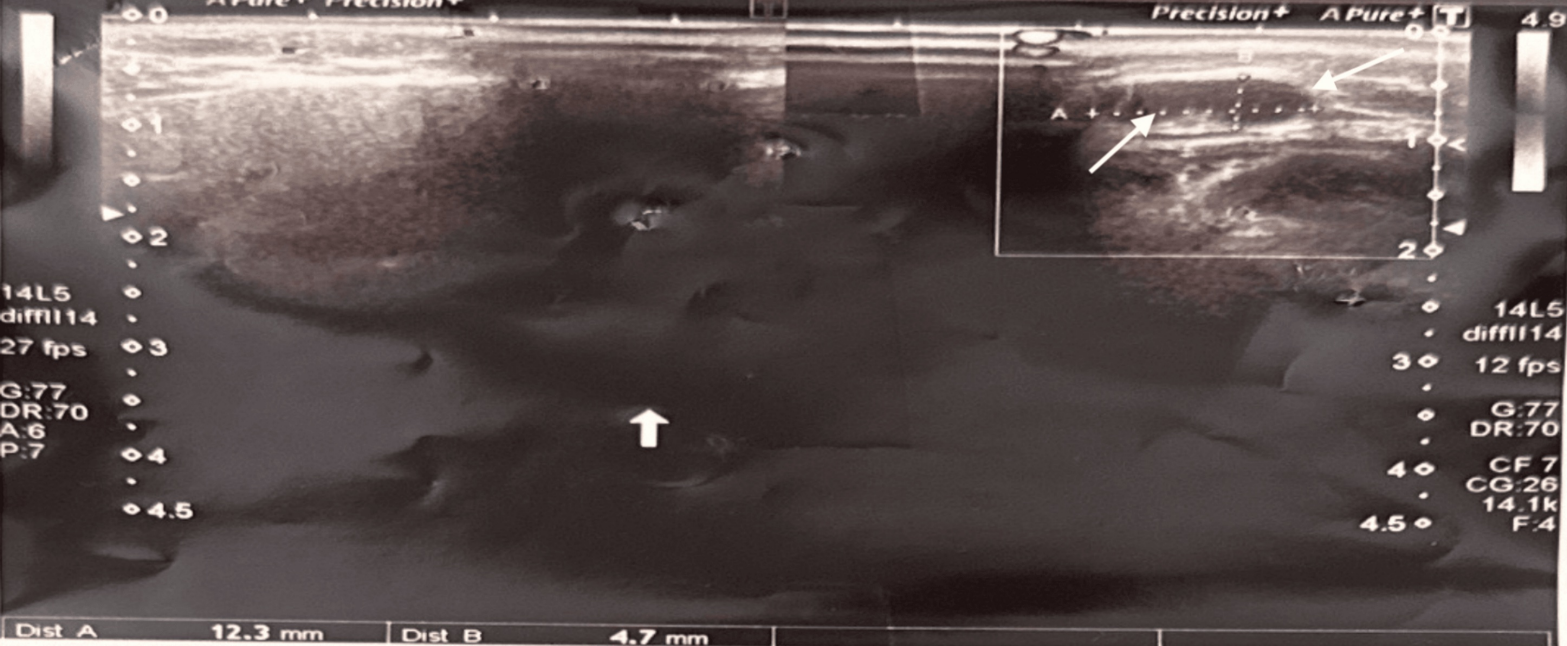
Ultrasound scan of a 22-year-old female showing a nuchal lymph node measuring 1.23 × 0.47 cm.
